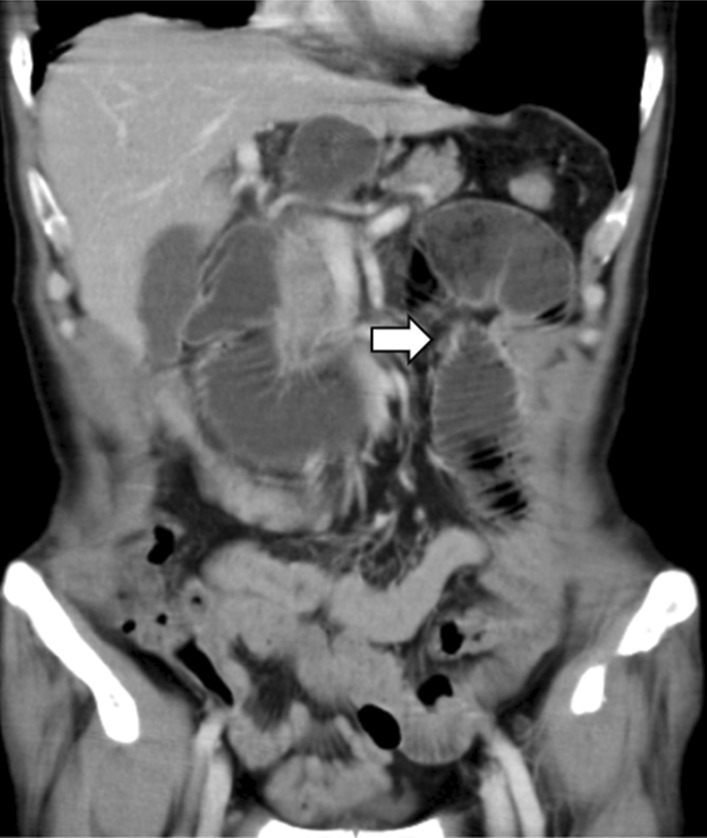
Contrast-enhanced CT. Enhanced CT shows dilation of the proximal bowel from jejunostomy (arrows: button jejunostomy site)
